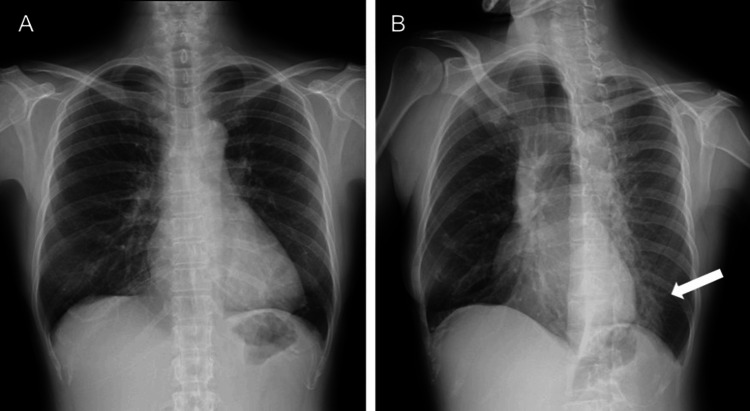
Chest plain radiographs at initial visit (A) Frontal view showing no significant decrease in lung field transparency. (B) Second oblique view showing a decrease in transparency in the left lower lung field (arrow).
